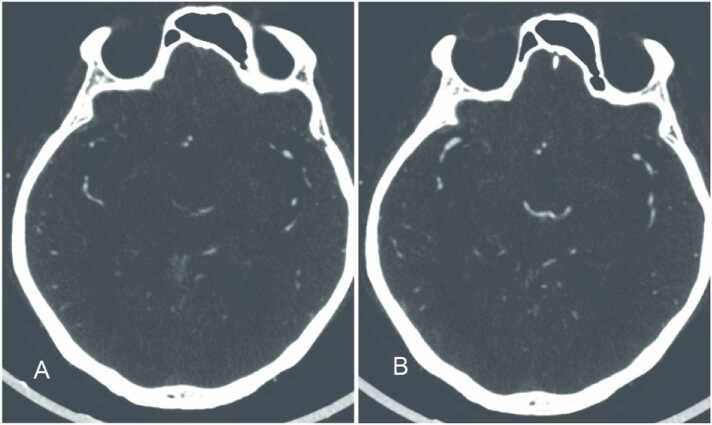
computed tomographic arteriography of Case 3 patient. CTA of Case 3 patient with AOP infraction show artery of Percheron.
